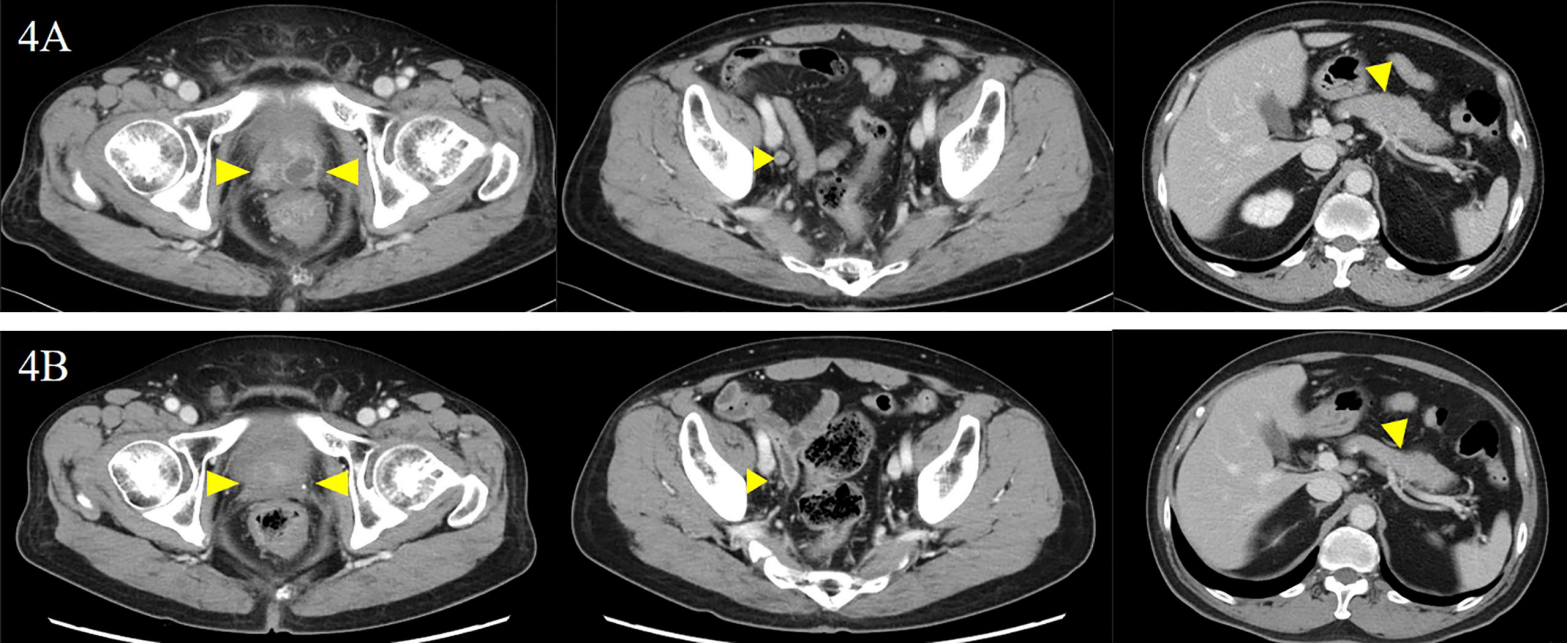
Local prostate lesion imaging including involved lymph nodes and pancreatic lesion before and after the administration of pembrolizumab. Contrast-enhanced computed tomography examination: (A) before the administration of pembrolizumab and (B) 5 months after the administration of pembrolizumab. There was complete remission of each lesion (yellow arrowheads).
